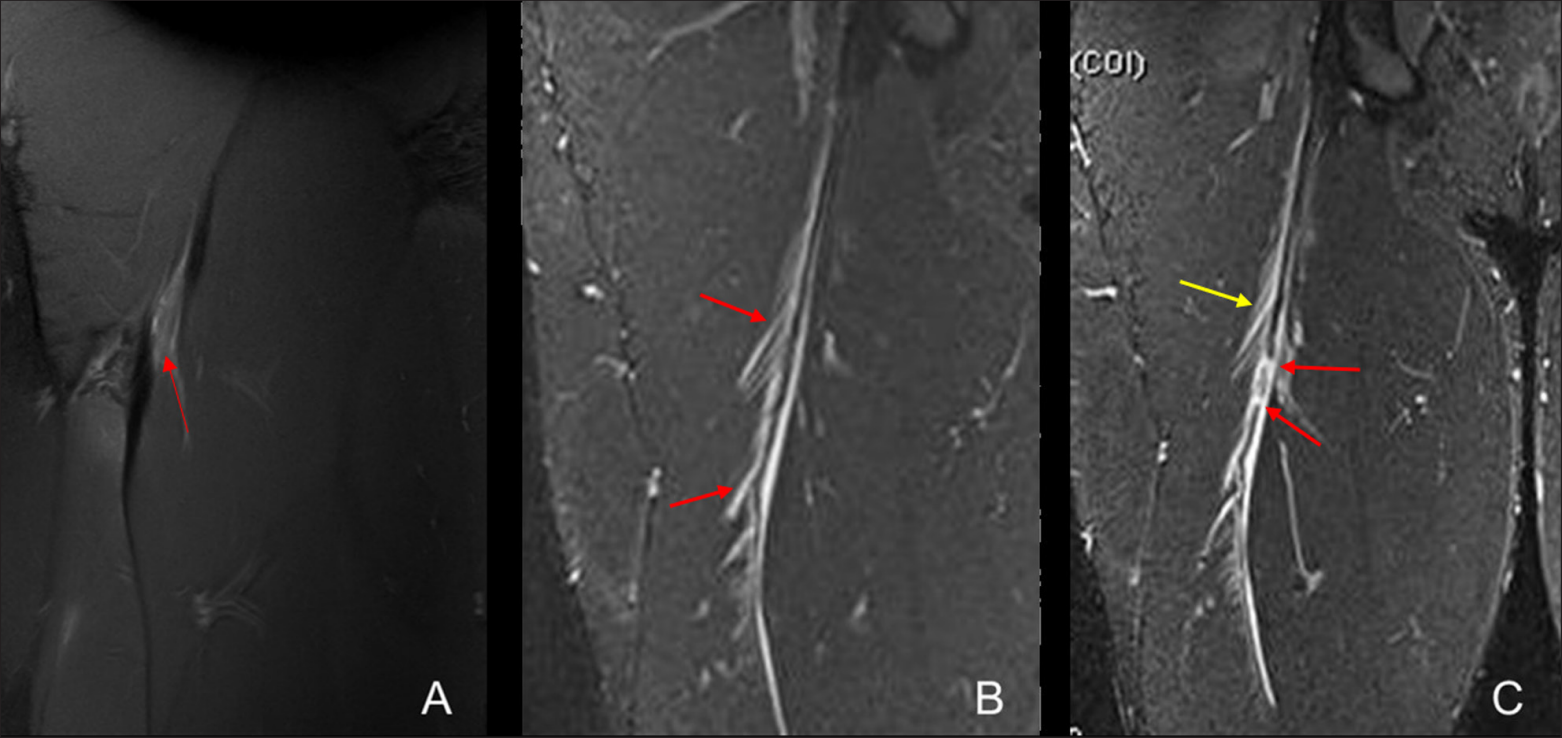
Coronal fat-suppressed proton density-weighted (a) and Short-Tau Inversion-Recovery (b and c) MRI of the right thigh of different patients. Complete tear of the free tendon in healing process (red arrow) (A), Myotendinous junction (MTJ) injury without tear of the BFLH connective tissue (red arrows) (B) and MTJ (yellow arrow) injury with mixed tear (transversal and longitudinal) of the central tendon (red arrows).
